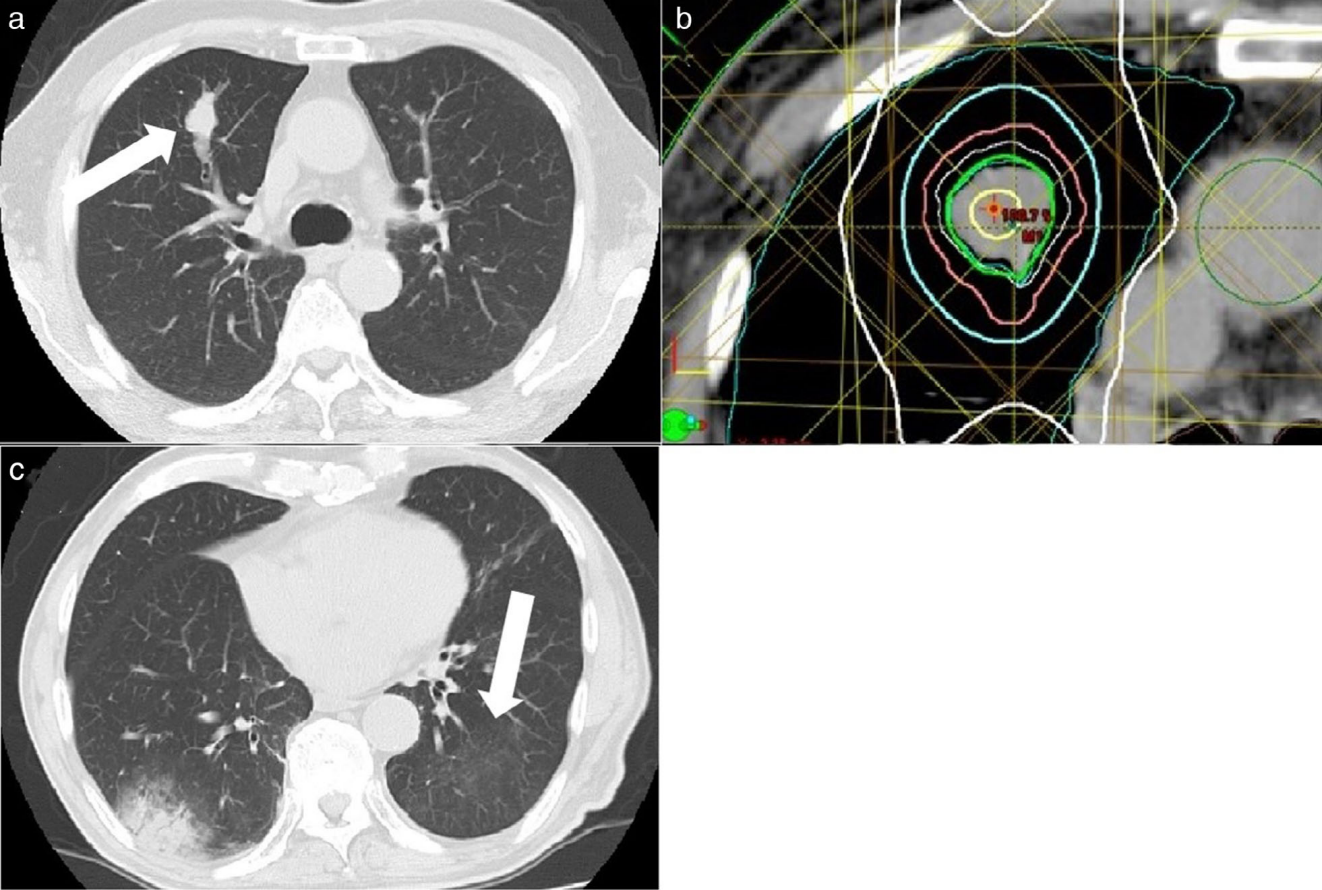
(a) Computed tomography (CT) shows primary lung cancer in the right upper lobe (arrow) in a male patient in his 80s. (b) He received three‐dimensional conformal radiotherapy; isodose lines of 100% (yellow), 95% (light green), 85% (light blue), 50% (white), and the planned target volume (pink) are shown. (c) After 13 courses of an anti‐PD‐1 antibody (24 months after thoracic radiation therapy), CT showed newly developed patchy opacity with air bronchograms in the irradiated area, which had spread to the low‐dose area and outside the irradiated area. Migration of lung infiltration with ground‐glass opacity in the left lung (arrow) was observed and diagnosed as organizing pneumonitis.
